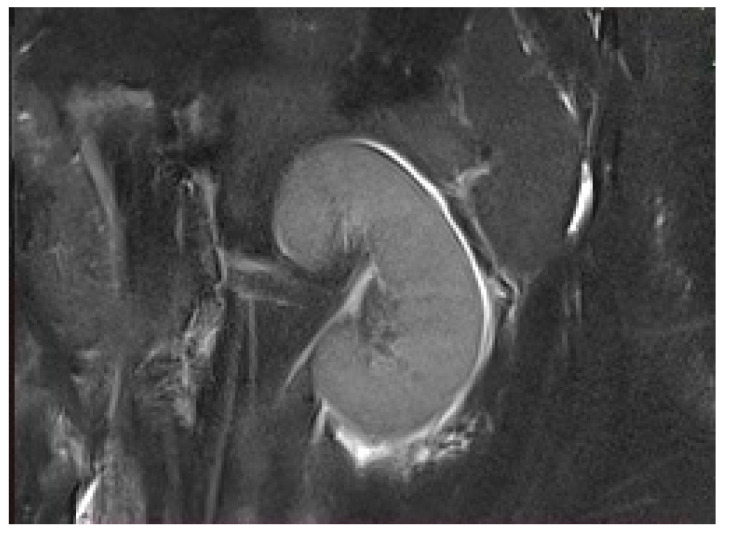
Example of kidney in T2-weighted Haste image.
